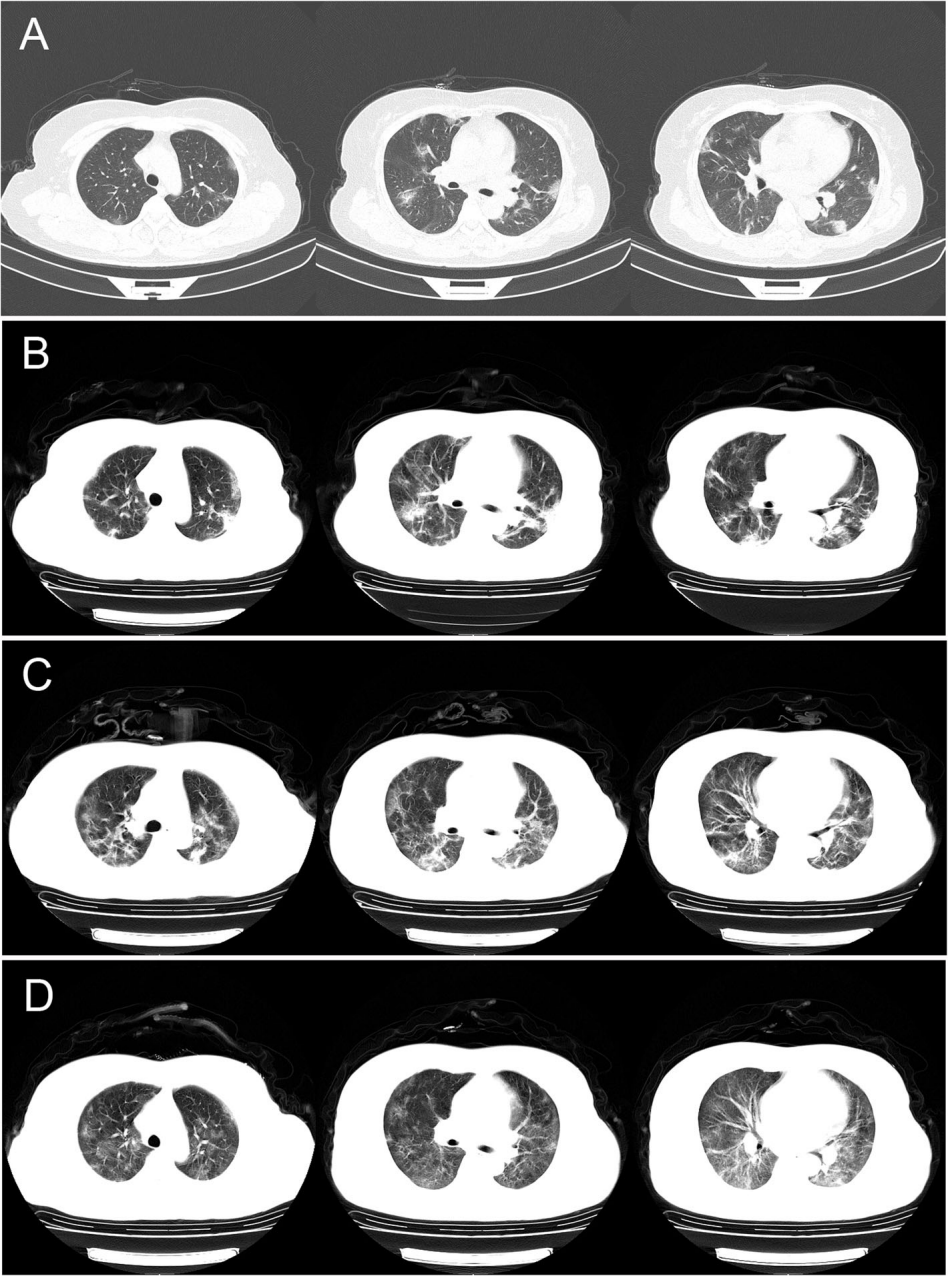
Chest CT of this patient. a Chest CT was obtained on January 16, 2020 (hospital day 1, illness day 7). Multiple patchy shadows and cord-like ground-glass opacity (GGO) under the pleura and bilateral lungs were observed. b Chest CT was obtained on January 19, 2020 (hospital day 4, illness day 10). The texture of the trachea and blood vessels in both lungs showed thickening. GGO increased, and the original GGO was consolidated. c Chest CT was obtained on January 23, 2020 (hospital day 8, illness day 14). The patchy lesions in both lungs were absorbed, and the fiber shadow increased in size. d Chest CT was obtained on January 30, 2020 (hospital day 15, illness day 21). The consolidation in the bilateral lungs was further absorbed. The fiber strands were reduced, and GGO increased slightly
